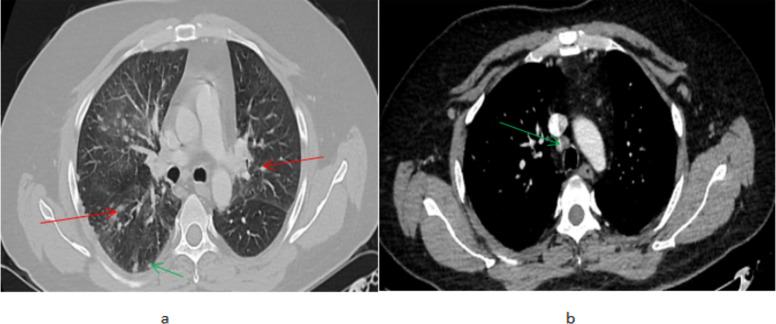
High-resolution axial chest CT (a) lung window showing bilateral different sized soft tissue attenuating pulmonary parenchymal nodules (red arrows) and a subpleural nodule on the right side (green arrow) (b) mediastinal window showing mildly enlarged right paratracheal mediastinal lymph node (green arrow)
